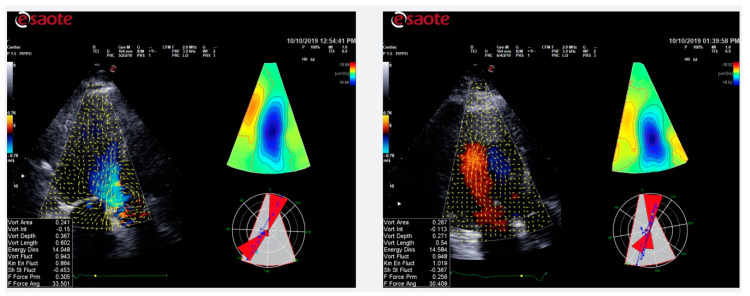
LV vortex analysis. During diastasis, left ventricular (LV) vortex maintains its rotary motion. Subsequently, at the moment of atrial contraction (late filling), a second vortex ring occurs, and the late filling jet combines with the residual early vortex ring. During the isovolumic contraction period, the vortex redirects blood toward the LV outflow tract, with formation of a large anterior vortex across the inflow–outflow region. HyperDoppler software by Esaote S.p.A on MyLab X8 eXP scanner.
